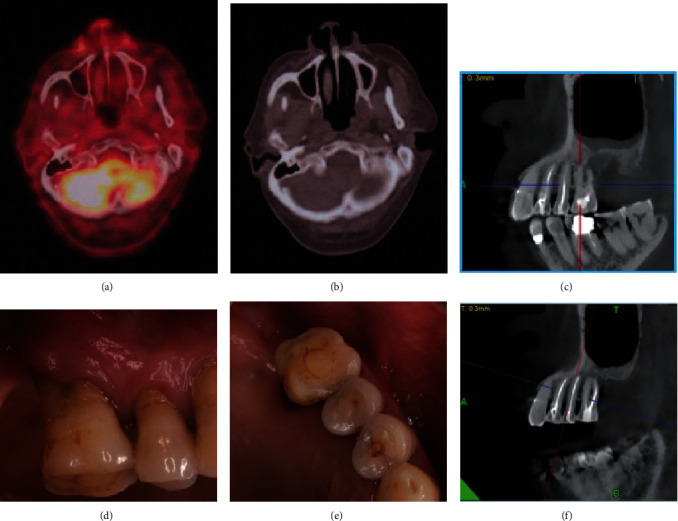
One month after the end of treatment, no distinct radioactive uptake in the left maxillary sinus was shown in transverse PET-CT images (a, b). CBCT image showed reduced substantial objects in the left maxillary sinus (c). Five years later, intraoral pictures showed healthy gingiva (d, e). CBCT showed that no substantial objects could be found in the left maxillary sinus (f).
